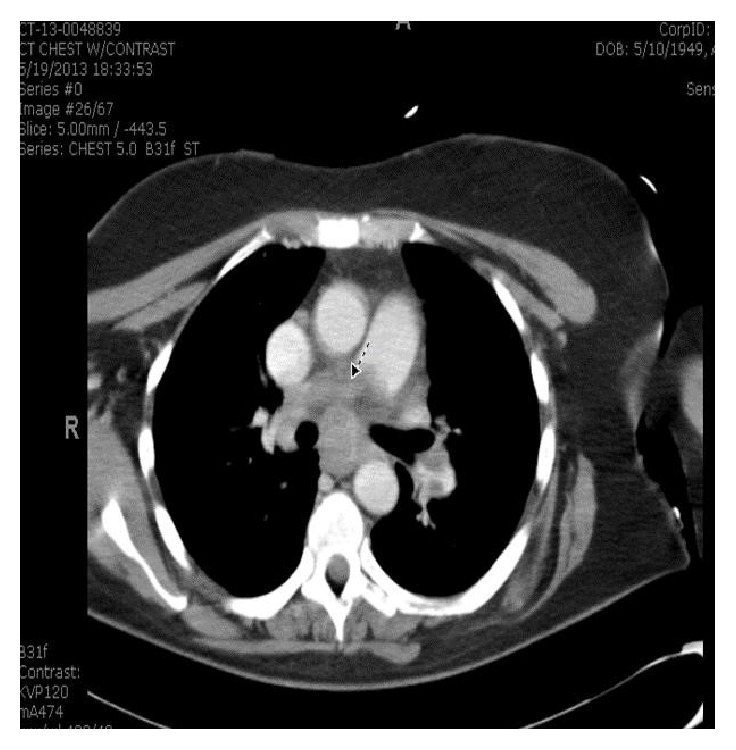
CT of the chest with arrow demarcating a saddle embolus.
